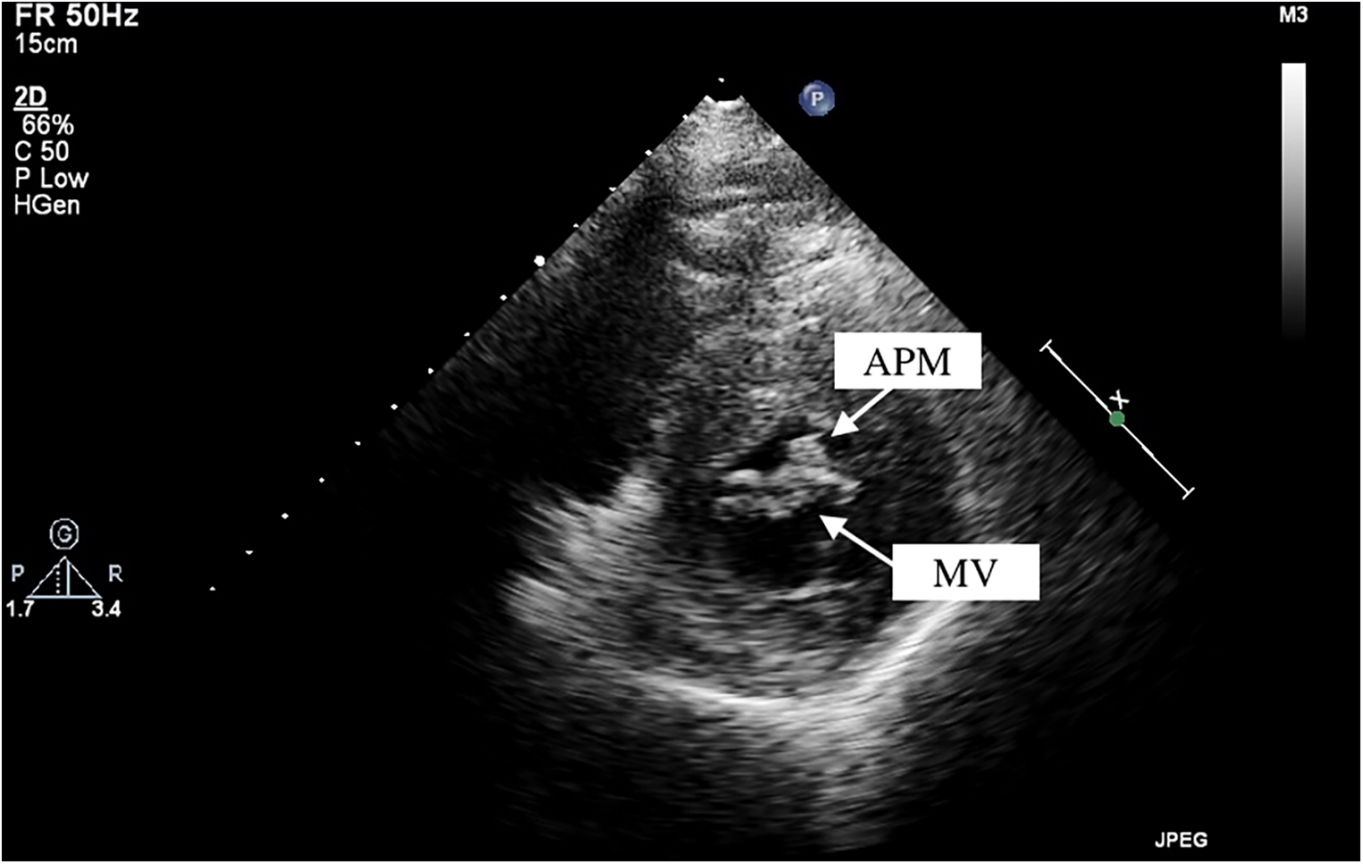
Anomalous papillary muscle (upper arrow) insertion into the mitral valve (lower arrow) visualized by transthoracic echocardiography. APM, anomalous papillary muscle; MV, mitral valve.
